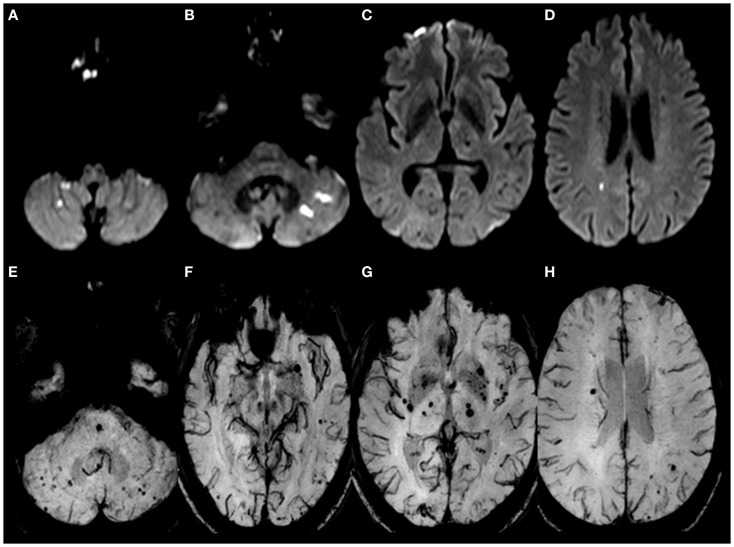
Brain MRI performed during the previous ischemic stroke 4 months earlier. (A–D) DWI showed restricted fluid diffusion in cerebellar hemispheres, left temporal cortex, right parietal deep white matter consistent with acute infarctions. (E–H) SWI-MRI (susceptibility weighted imaging MRI) showed multiple cerebral microbleeds, particularly in the deep gray matter and posterior fossa, possibly due to hypertensive vasculopathy.
